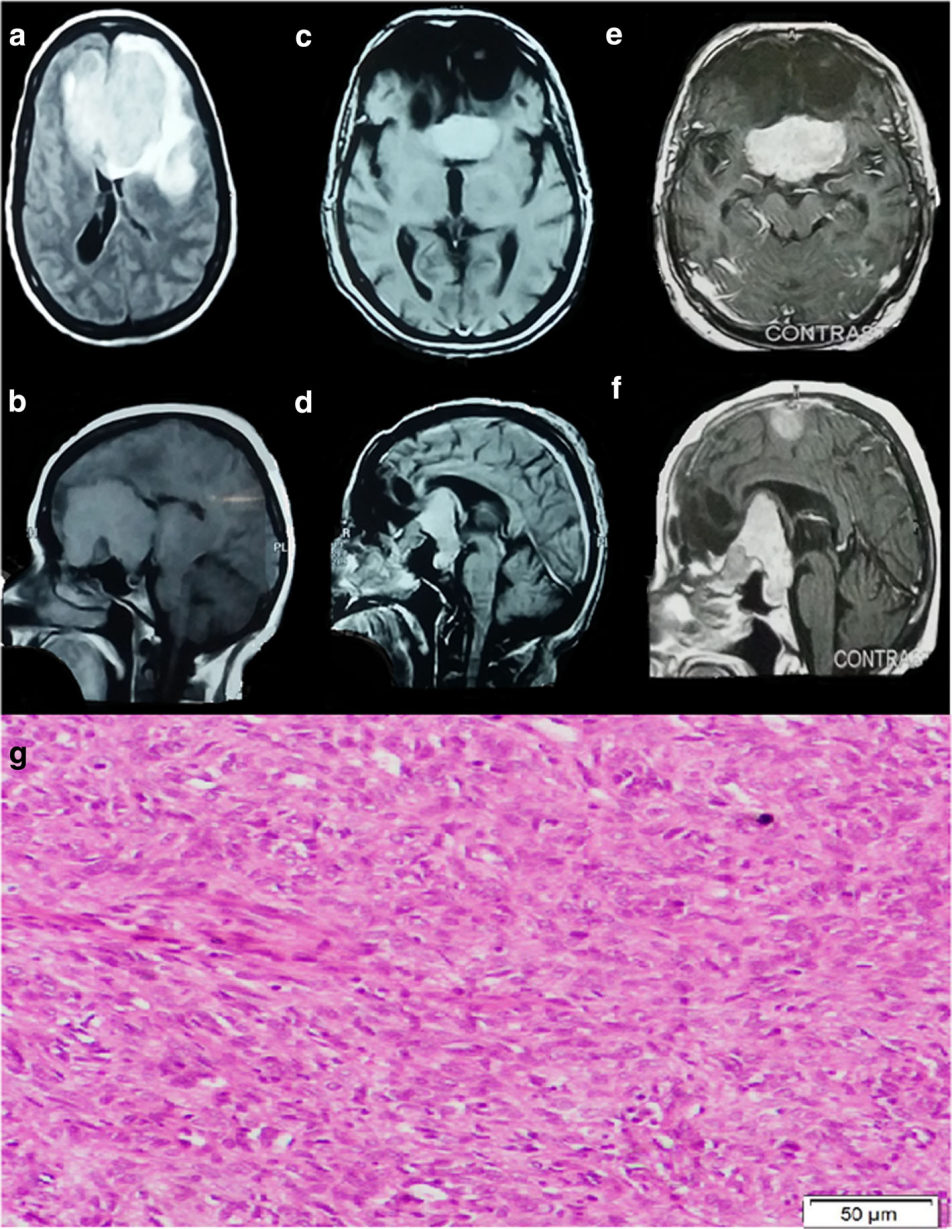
An olfactory groove meningioma. a Preoperative image, MRI with contrast (axial) showed mass lesion occupied the frontal region. b MRI with contrast (sagittal) demonstrated mass lesion arising from the floor of the anterior cranial fossa. The tumor was surrounded by marked edema. Geriatric Scoring System (GSS) score = 12 C). Four years postoperative MRI with contrast, c axial and d sagittal views showed recurrence of the tumor with extension into the third ventricle. The patient family refused surgery. Geriatric Scoring System (GSS) score = 11. e Six year postoperative MRI with contrast. e Axial cut represented progressive large tumor widening and splaying the arteries of the circle of Willis and the cerebral peduncles. f Sagittal view revealed the tumor was abutting the optic chiasma and reached the anterior and inferior aspect of the 3rd ventricle. It sent a distal metastasis into the left frontal cortical region. The family again refuse the surgery, and the patient were at high risk with repeated convulsion, and totally blind. g The slide represented an atypical meningioma WHO grade II. At power 10× the slide showed hypercellular tumor tissue formed of whorls of pleomorphic meningothelial cells showing pleomorphic and moderately hyperchromatic nuclei with frequent mitotic figures
